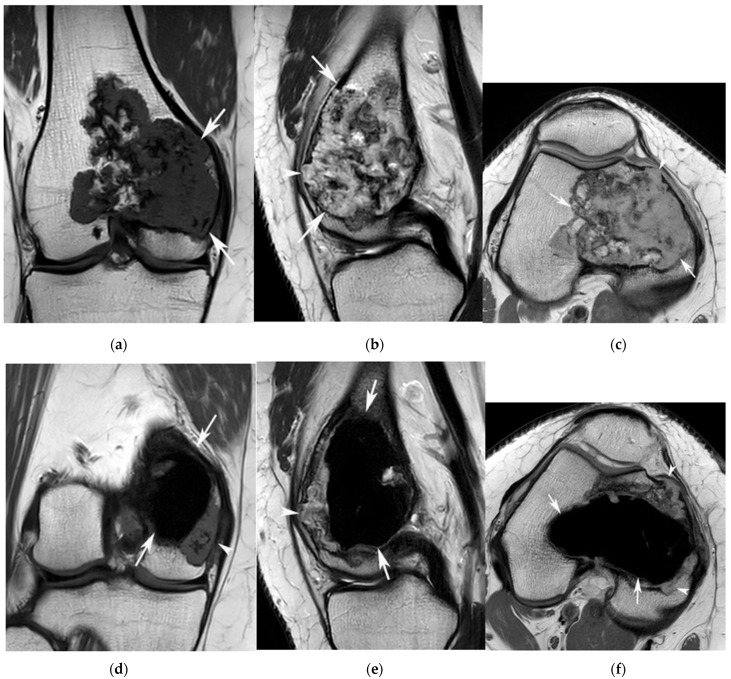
A 28-year-old female with an ACT in the right distal femur. (a) Coronal T1W TSE, (b) sagittal T2W FSE and (c) axial PDW FSE MR images show a lobular chondral tumour (arrows) extending into the epiphysis of the femur associated with areas of cortical breach (arrowheads (b,c)). Repeat imaging 9 months later. (d) Coronal T1W TSE, (e) sagittal T2W FSE and (f) axial PDW FSE MR images show the cementoma (arrows) following curettage and multifocal areas of local recurrence (arrowheads).
